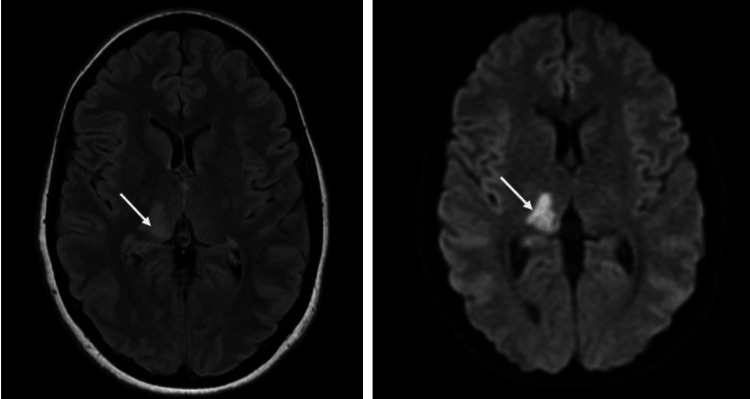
MRI axial view of the brain The left image is the T2/FLAIR, and the right image is the DWI, showing the high signal intensity indicated by arrows DWI: diffusion-weighted imaging; FLAIR: fluid-attenuated inversion recovery; MRI: magnetic resonance imaging
